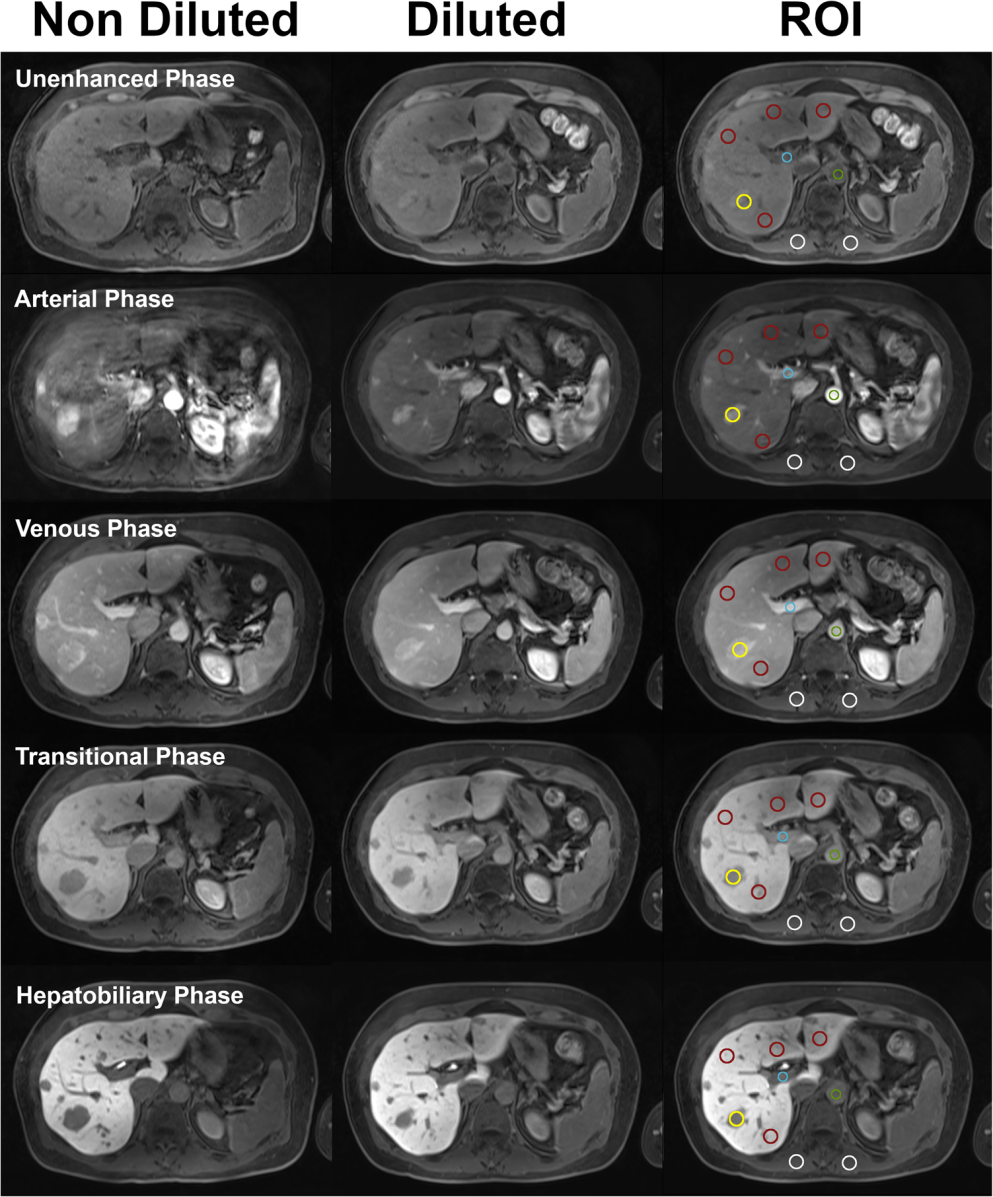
Three columns of axial liver MR images showing non-diluted and diluted injections in the same patient. The last column shows the regions of interest (ROI) as measured in the five specified locations: aorta (green), portal vein (blue), liver parenchyma (red), focal liver lesion (yellow), and paraspinal muscle (white) in all phases
